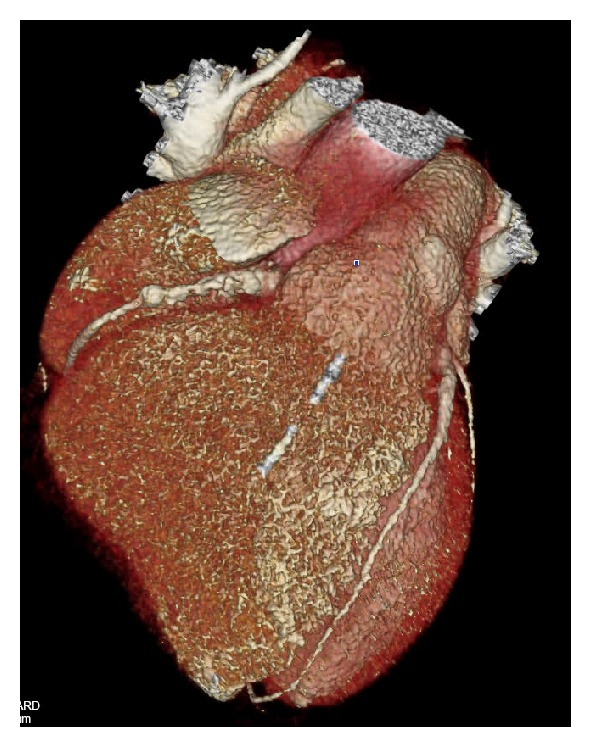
CTA Volume-Rendered Image demonstrating coronary artery aneurysms.
